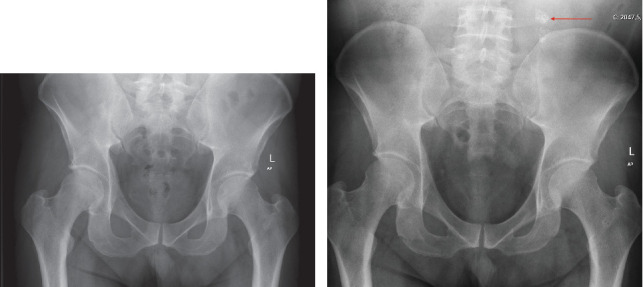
(a) Initial pelvic radiograph taken in the emergency room. (b) Second pelvic radiograph taken in the radiology department; red arrow showing the calcifications.
